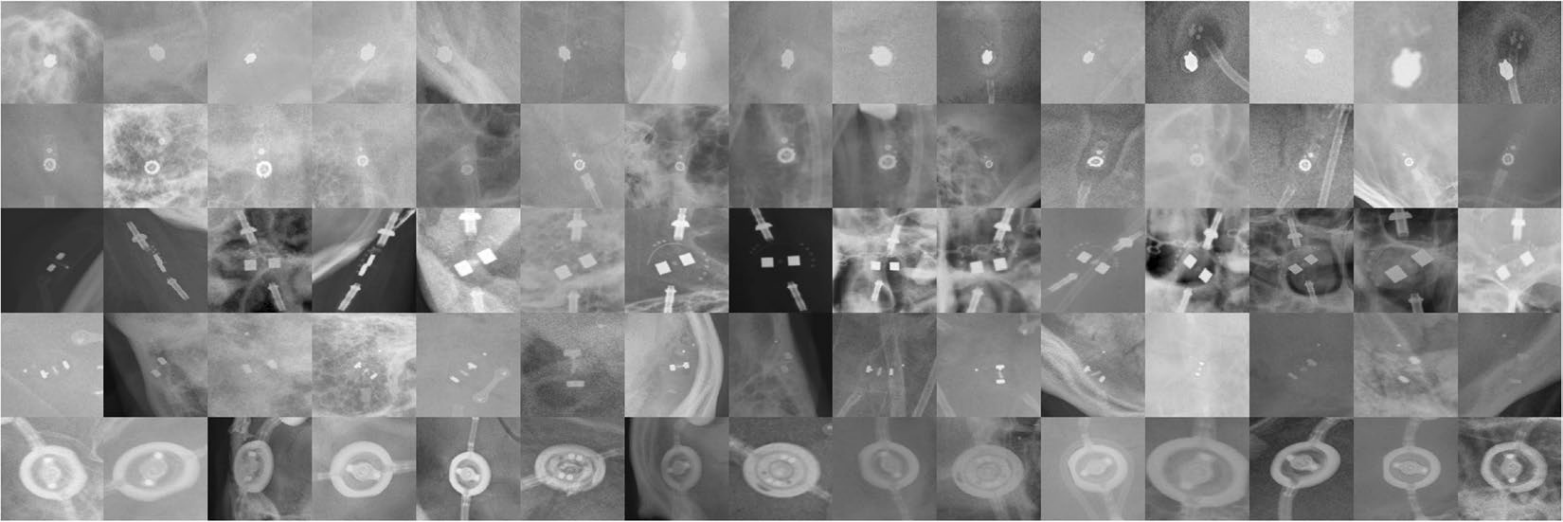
Examples of CSF-SV X-ray valve images in our dataset. Each row shows 15 random samples for each of the 5 classes used. From the top, row 1: *Medtronic Strata II - NSC* (n = 164); row 2: *Codman-Hakim* (n = 106); row 3: *Sophysa Polaris SPV* (n = 82); row 4: *Codman-Certas* (n = 42); row 5: *Miethke ProGAV* (n = 22). In parentheses, the number of samples contained in the dataset. The dataset contains all of the shunt valves brands currently used in US.
